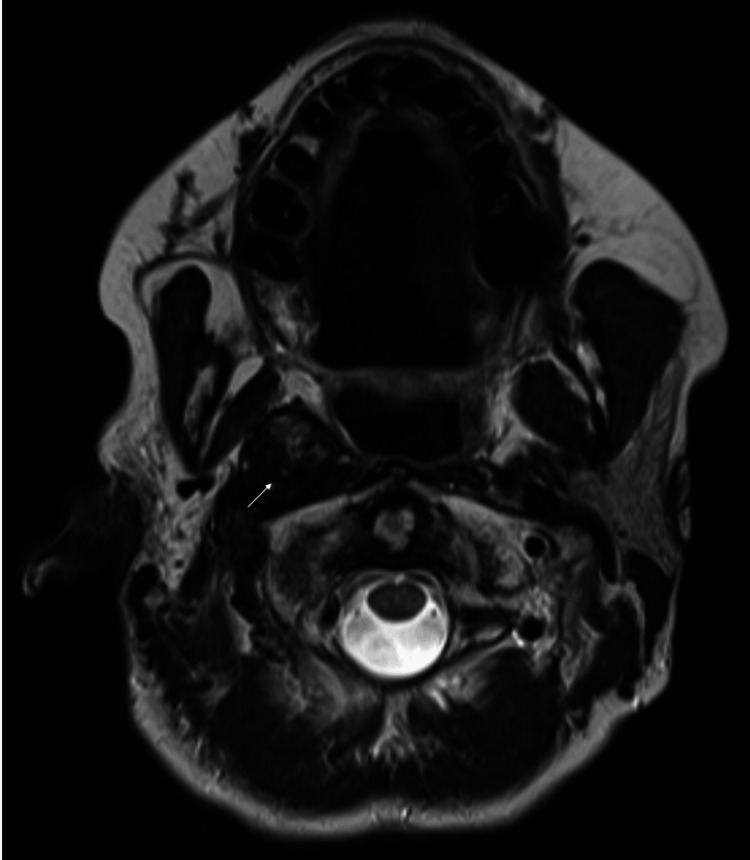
T2-weighted MRI of prior radiation bed (arrow) in December 2021 demonstrating no increase in size from the previous scan
